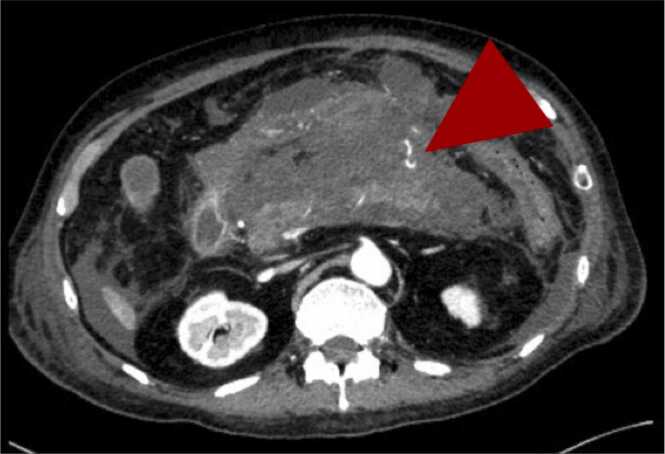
Contrast-enhanced abdominal Computed tomographic (CT) images reveal an extensive area of inflammation surrounding the pancreas. Extravasation of contrast material, indicated by arrows, suggests active bleeding.
